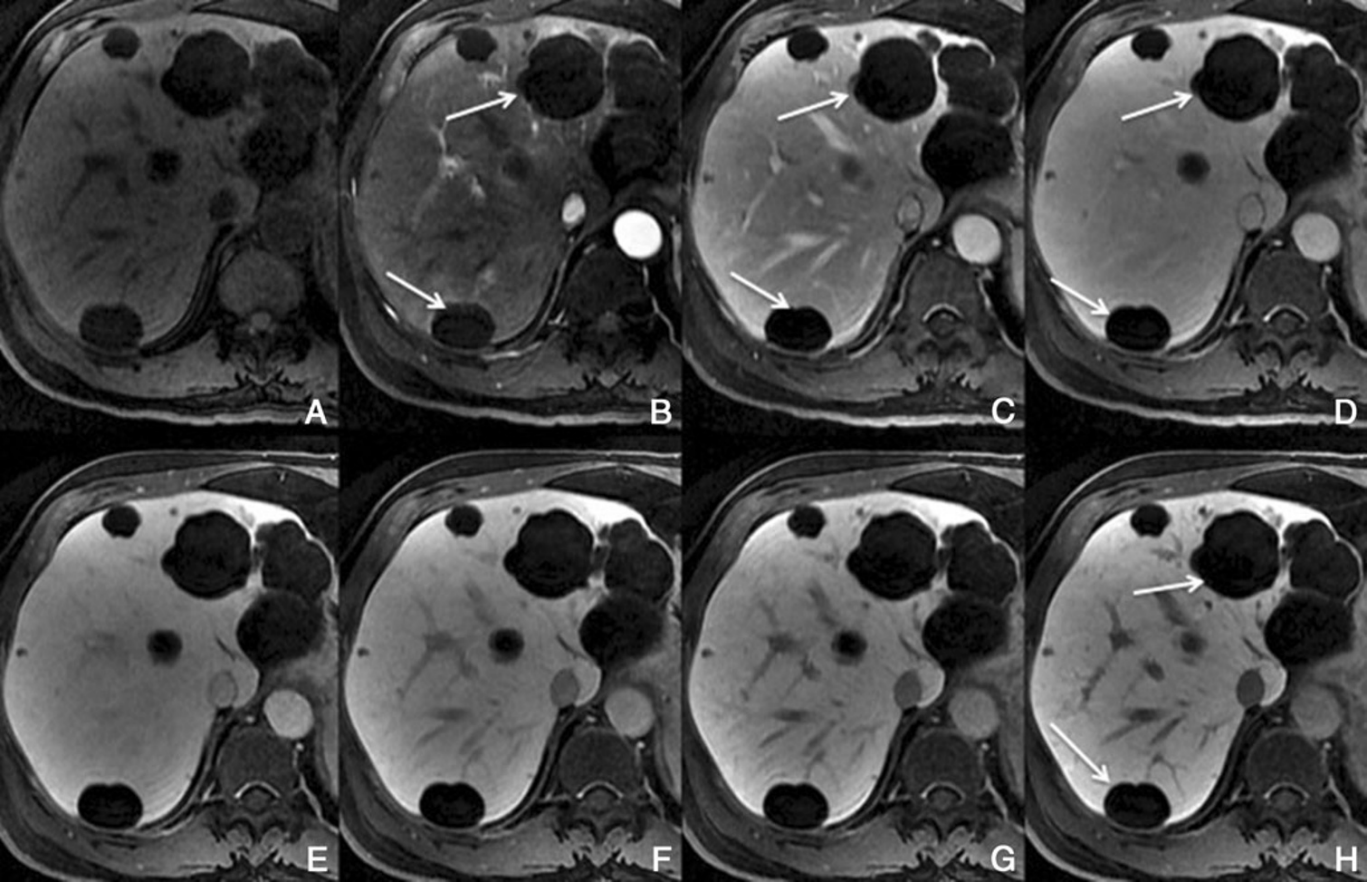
Multiple hepatic cysts, in a 47-year-old woman, with no liver disease. a Pre-contrast, b arterial, c PVP, d 3 min, e 5 min, f 8 min, g 10 min, h HCP. Multiple rounded hypointense lesions (*arrows*), with no enhancement during all the phases. These lesions were also markedly hyperintense in T2-weighted imaging (not shown)
